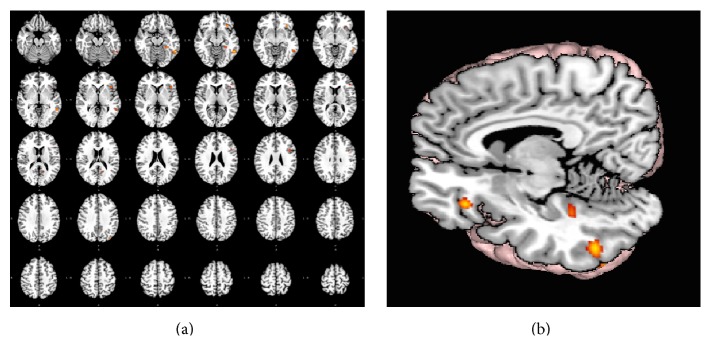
Functional connectivity map of BA20 by Meta-Analytic Connectivity Modeling. (a) Transversal descending cuts of the brain MRI template. Left hemisphere appears on the left side (neurological convention). Clusters of activation are color coded for statistical significance from dark blue (lowest) to red (highest). (b) 3D volumetric rendition of the brain showing activation on the left hemisphere surface. Red color zone identifies BA20. Deep and midline activations are shown.
